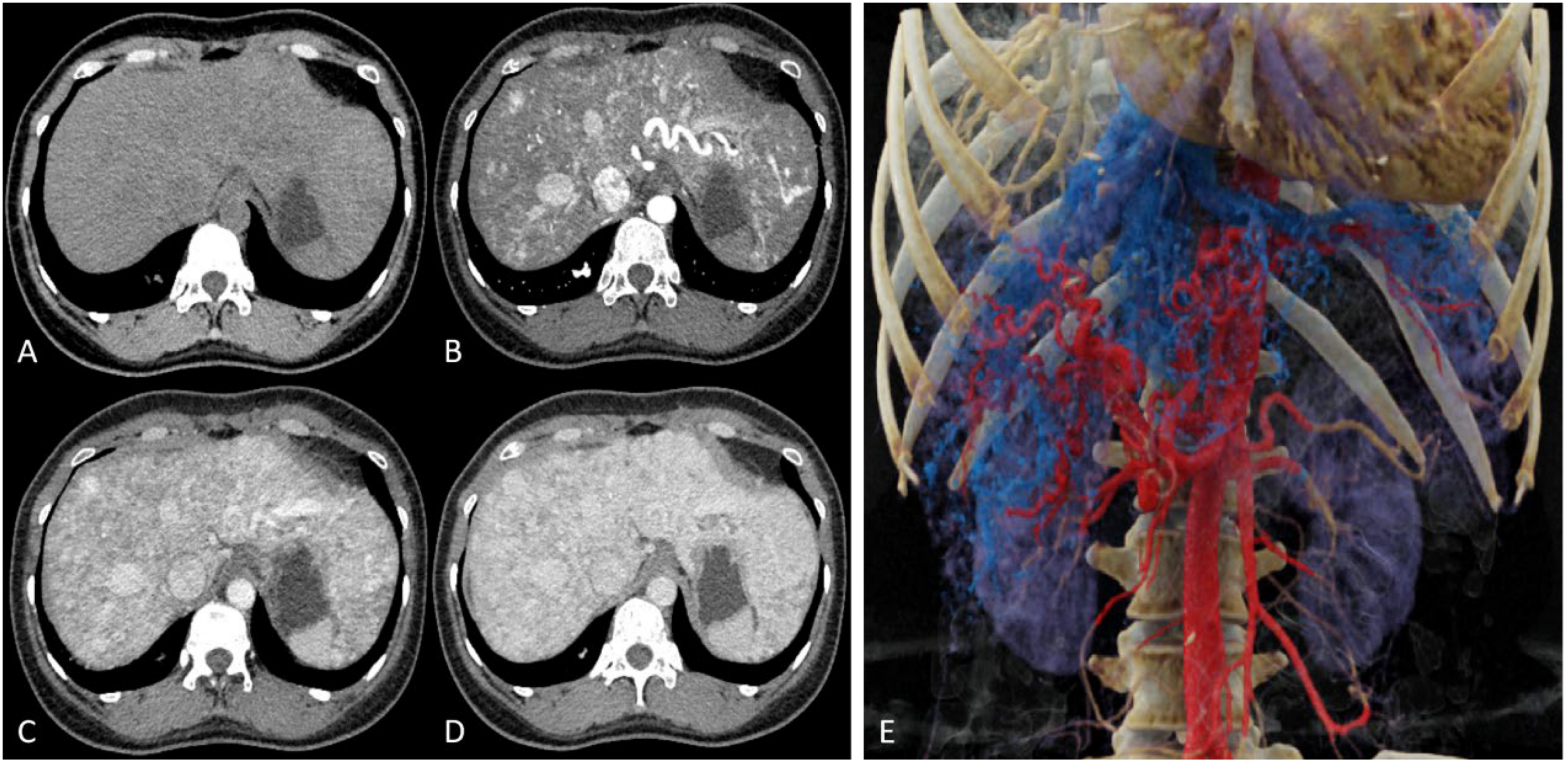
(A) Plain CT reveals multiple tortuous, enlarged low-density vascular shadows in the liver. (B) In the arterial phase, multiple tortuous and thickened arteries and veins can be seen within the liver. (C) The portal venous phase and (D) equilibrium phase indicate uniform liver enhancement with mildly dilated intrahepatic bile ducts. (E) 3D reconstruction images from CTV display tortuous, enlarged arteries and veins, along with dilated hepatic veins and superior mesenteric veins.
